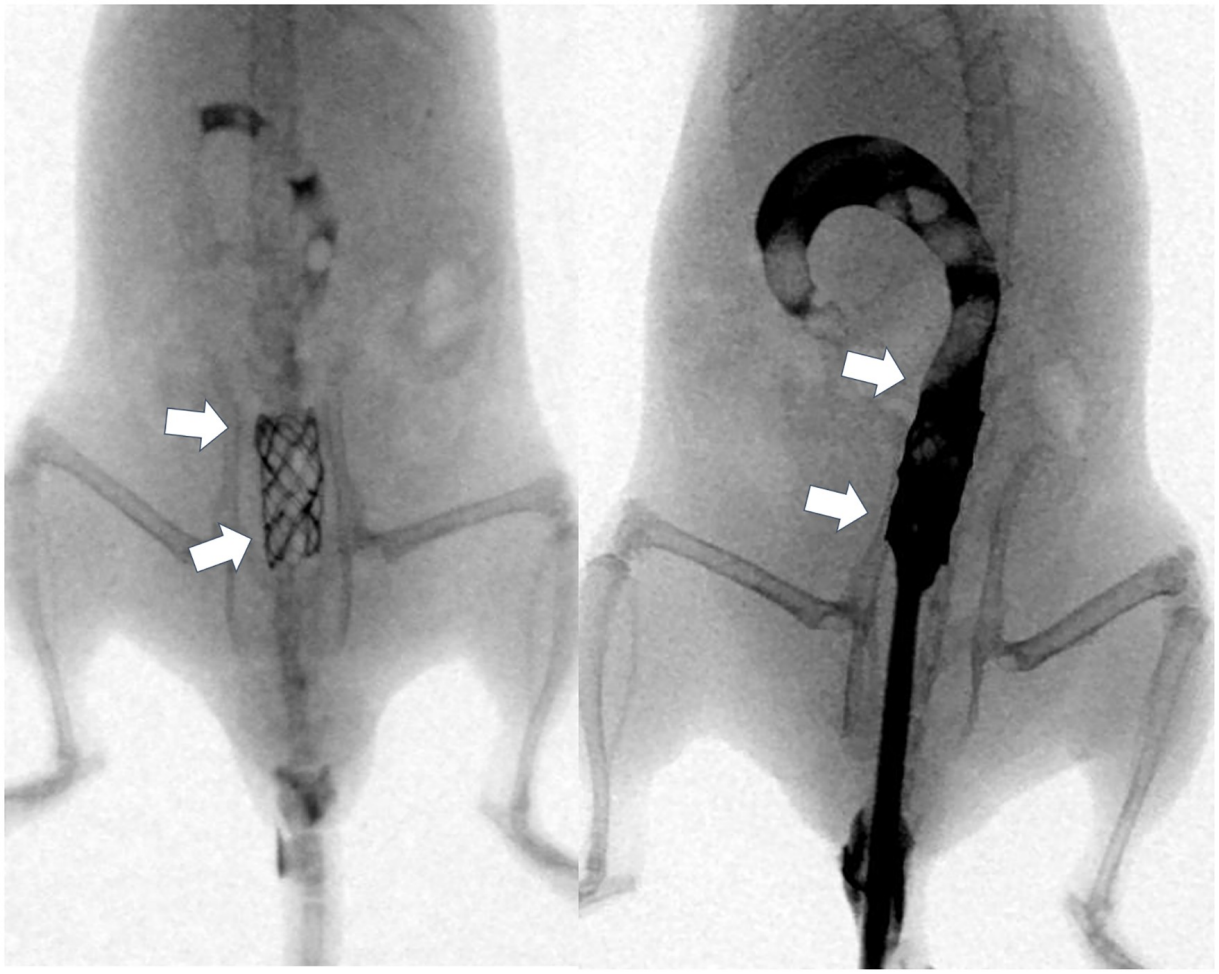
Representative radiographic images showing the stent in the sigmoid colon at the level of the pelvis. [Arrows = stented sigmoid colon at the level of the pelvis].
